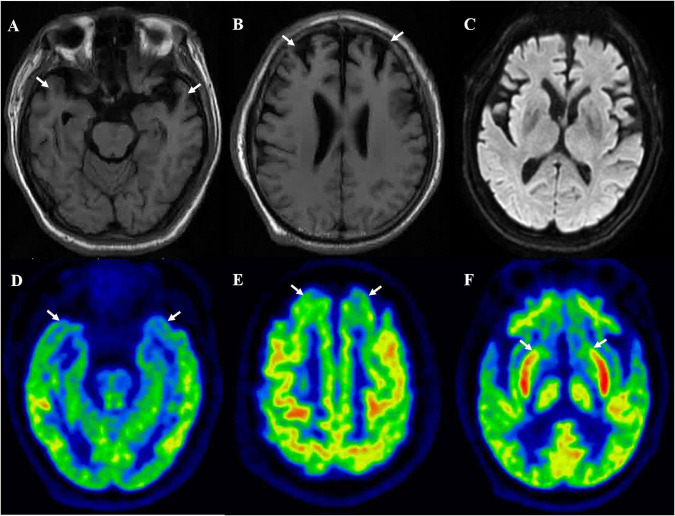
Brain MRI suggested bilateral frontal (B) and temporal (A) lobes atrophy, and no hyperintensity was detected in diffusion-weighted imaging (C). [^18^F]-FDG-PET/MRI suggested bilateral frontal lobe (E), temporal lobe (D), and caudate nucleus (F) hypometabolism.
